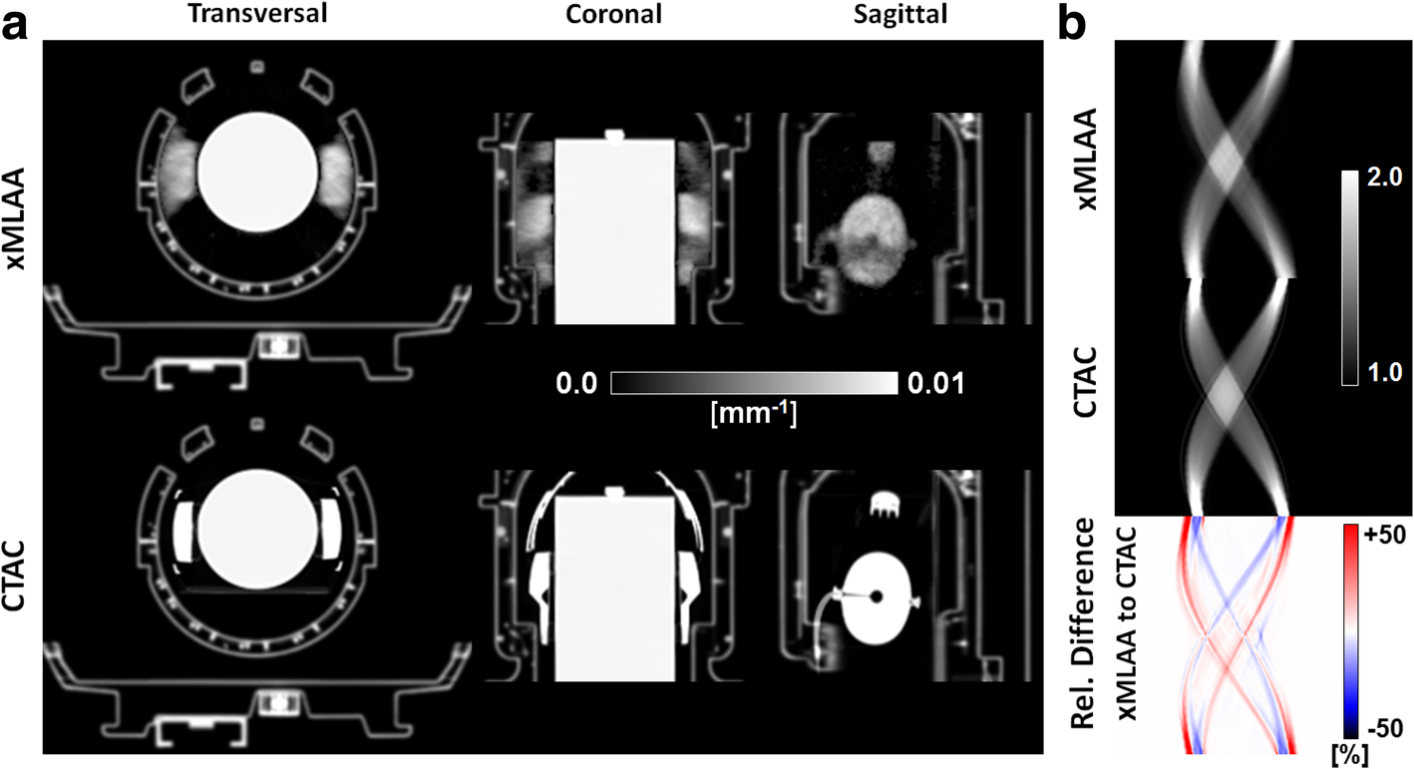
Results for the HP experiment: attenuation. a Transversal, coronal, and sagittal views of the xMLAA- and CT-based attenuation maps. b Attenuation correction factors (ACFs) of the headphones only. The sinograms correspond to the same transversal plane as shown in (a)
